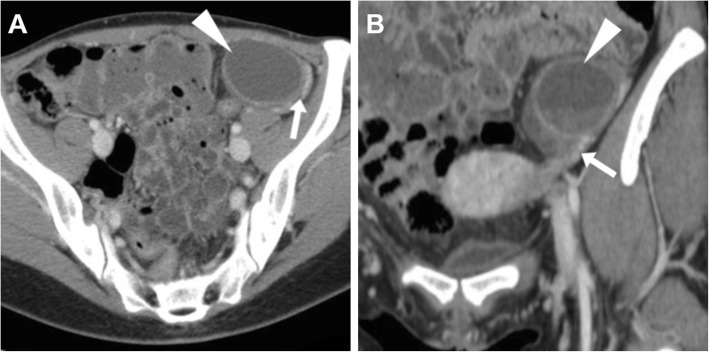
Uncomplicated, symptomatic follicular ovarian cyst causing left lower quadrant pain. Axial (a) and coronal (b) CT images show a 4 × 3 cm cystic mass (arrowheads) with homogeneous fluid attenuation, laterally displaced in the left hemipelvis. Identification of the ovarian vessels in the suspensory ligament (arrows) helps in confirming the adnexal origin of the cyst
